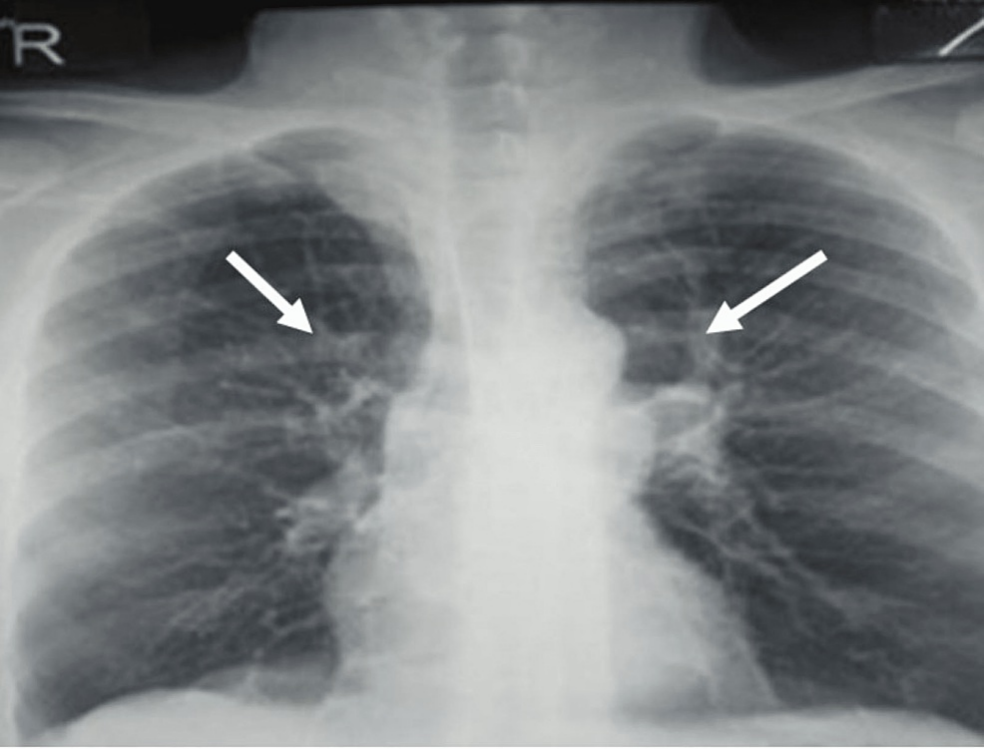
Chest X-ray showing linear opacity associated with retraction signs in the hilar regions.
